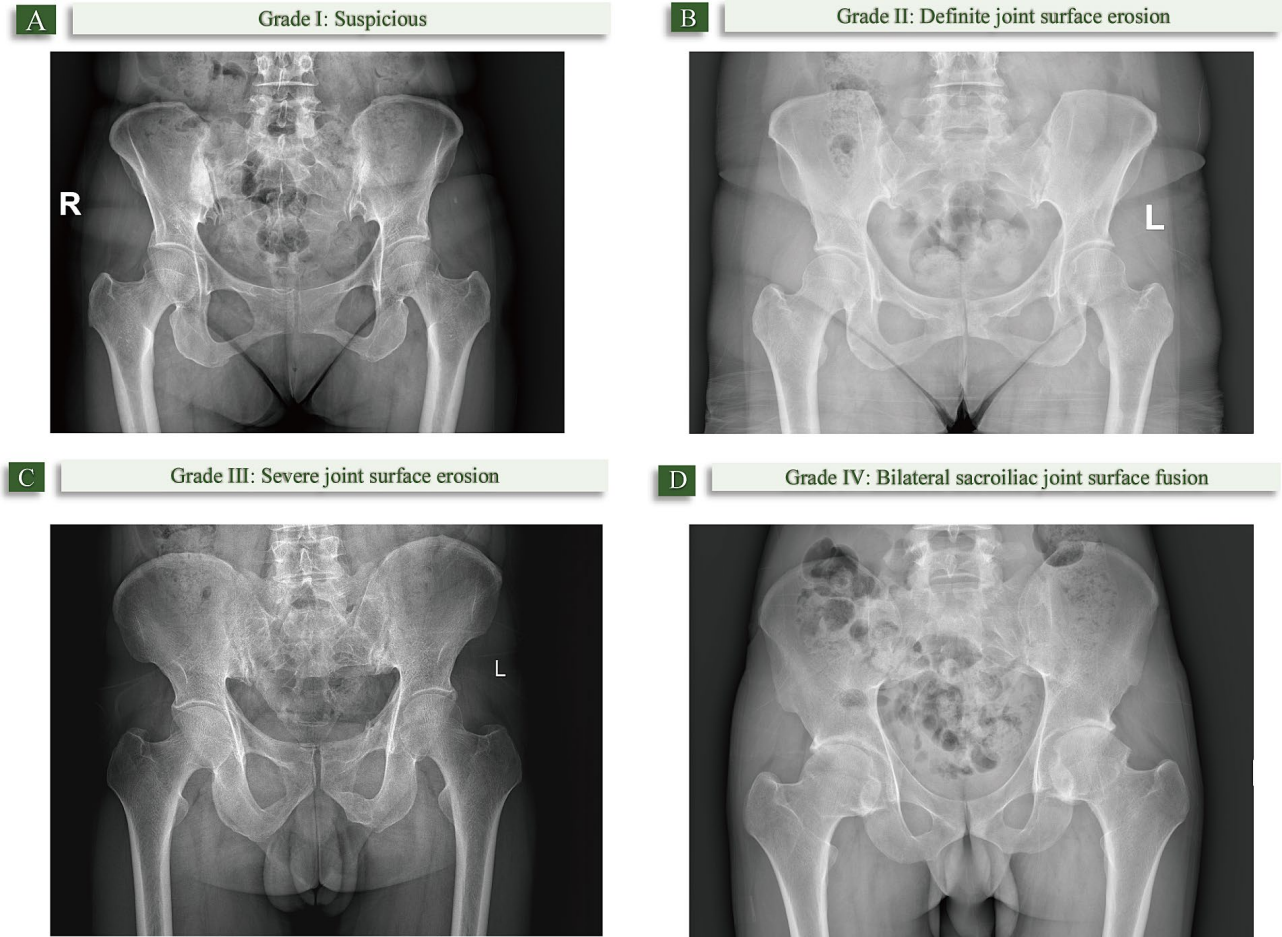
The degree of sacroiliitis on X-ray. (A) Grade I: Suspicious (joint surface roughness, small cystic changes, slight sclerosis); (B) Grade II: Definite joint surface erosion without significant joint space changes; (C) Grade III: Severe joint surface erosion, pseudo-widening/narrowing of joint space, or partial fusion; (D) Grade IV: Bilateral sacroiliac joint fusion.
